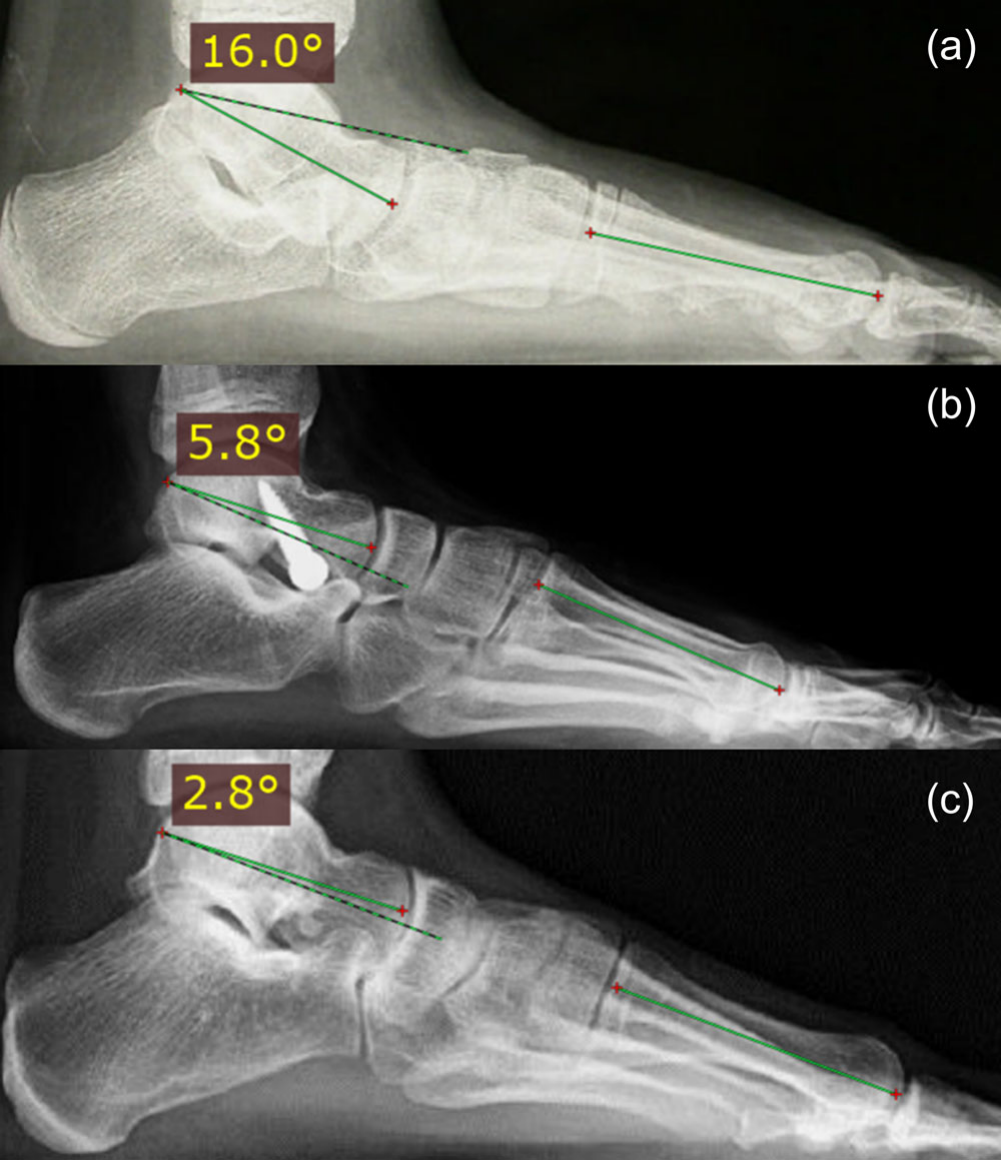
Talar–first metatarsal angle (Meary's angle), between the lines drawn along the long axis of the talus and the first metatarsal in lateral radiographic view, before correction (a), with the screw implanted (b) and 11 years after screw removal (c).
